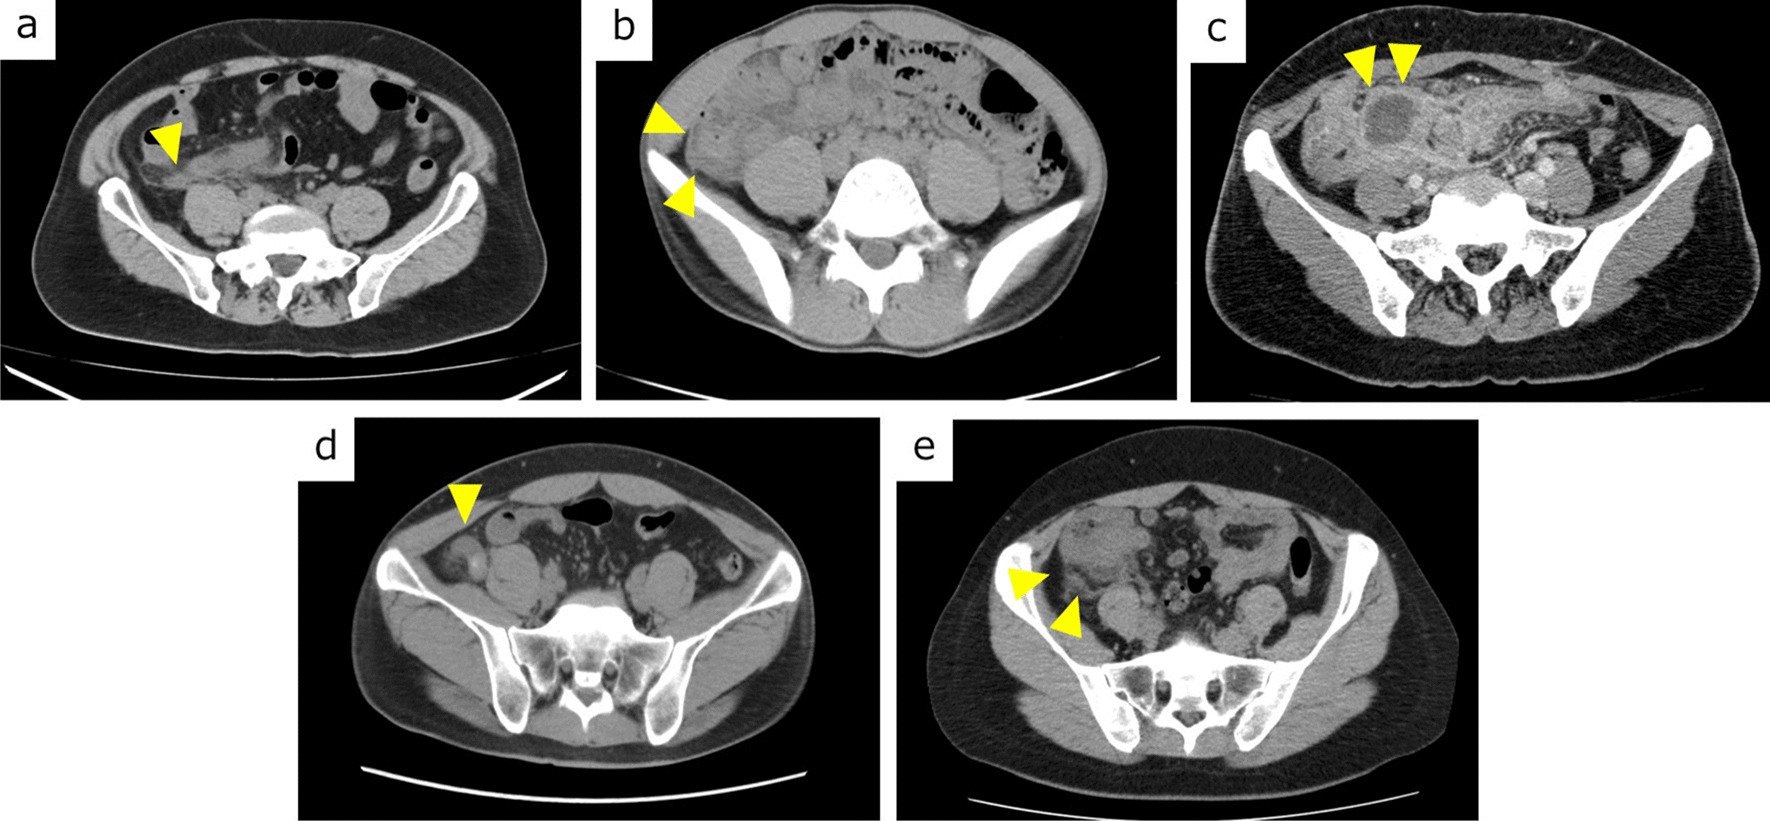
Computed tomography images. a, b Localized fluid collection around the appendix and cecum (arrowheads). c Localized abscess formation (arrowheads). d, e Swelling of the appendix and fat stranding are observed; however, no fluid collection is observed (arrowheads)
